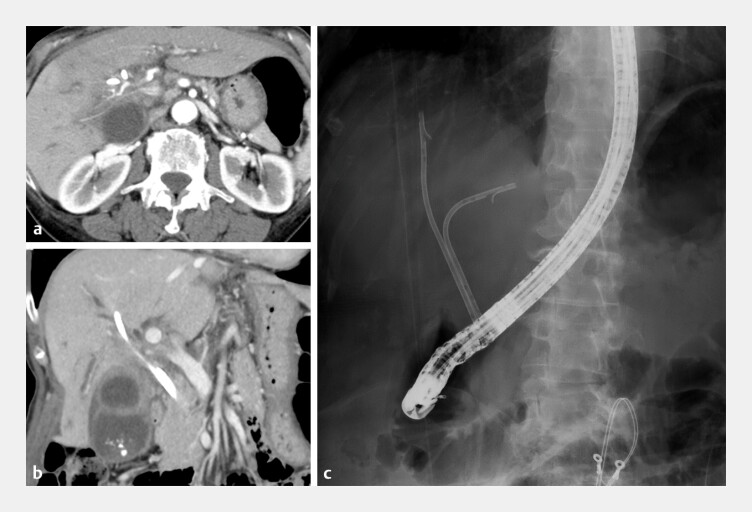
Computed tomographic (CT) and fluoroscopic images.
